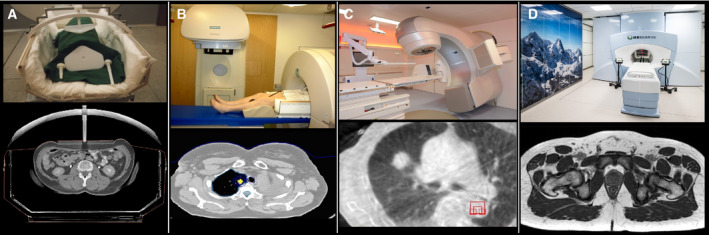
Development of image guidance in SBRT. (A) External stereotactic coordinates of the stereotactic body frame, (B) in‐room CT, (C) integrated CBCT, (D) integrated MRI imaging.
